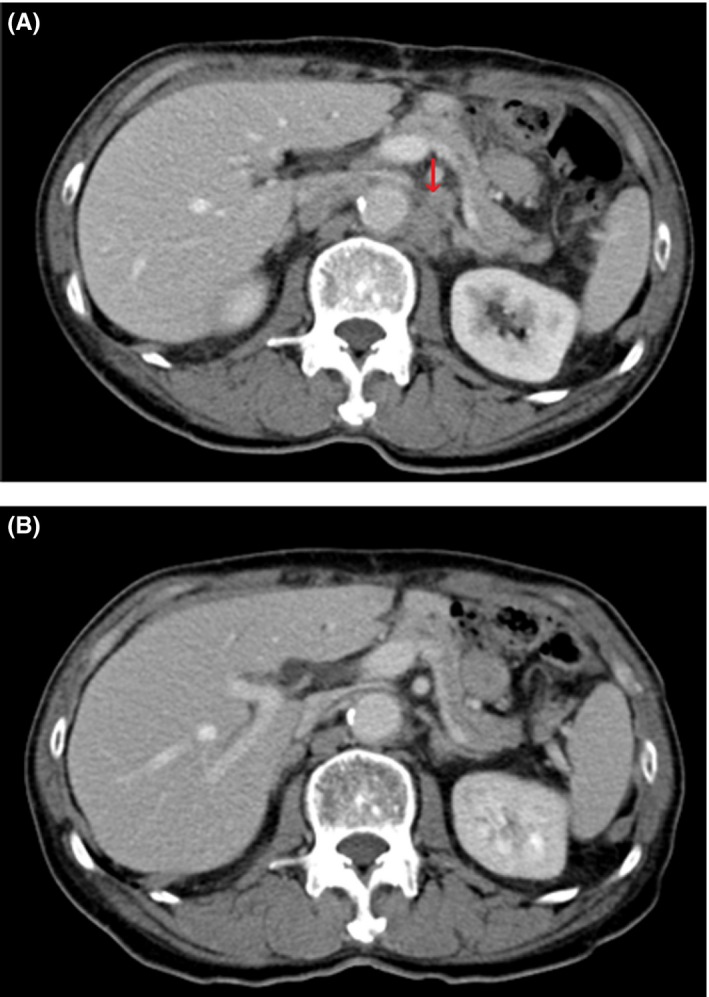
Enhanced computed tomography scan findings in case 2. A, Lymph node recurrences at the para‐aortic region were identified 4 months after surgery (arrow). B, Para‐aortic lymph node recurrences showed remarkable regression after trastuzumab plus capecitabine therapy
